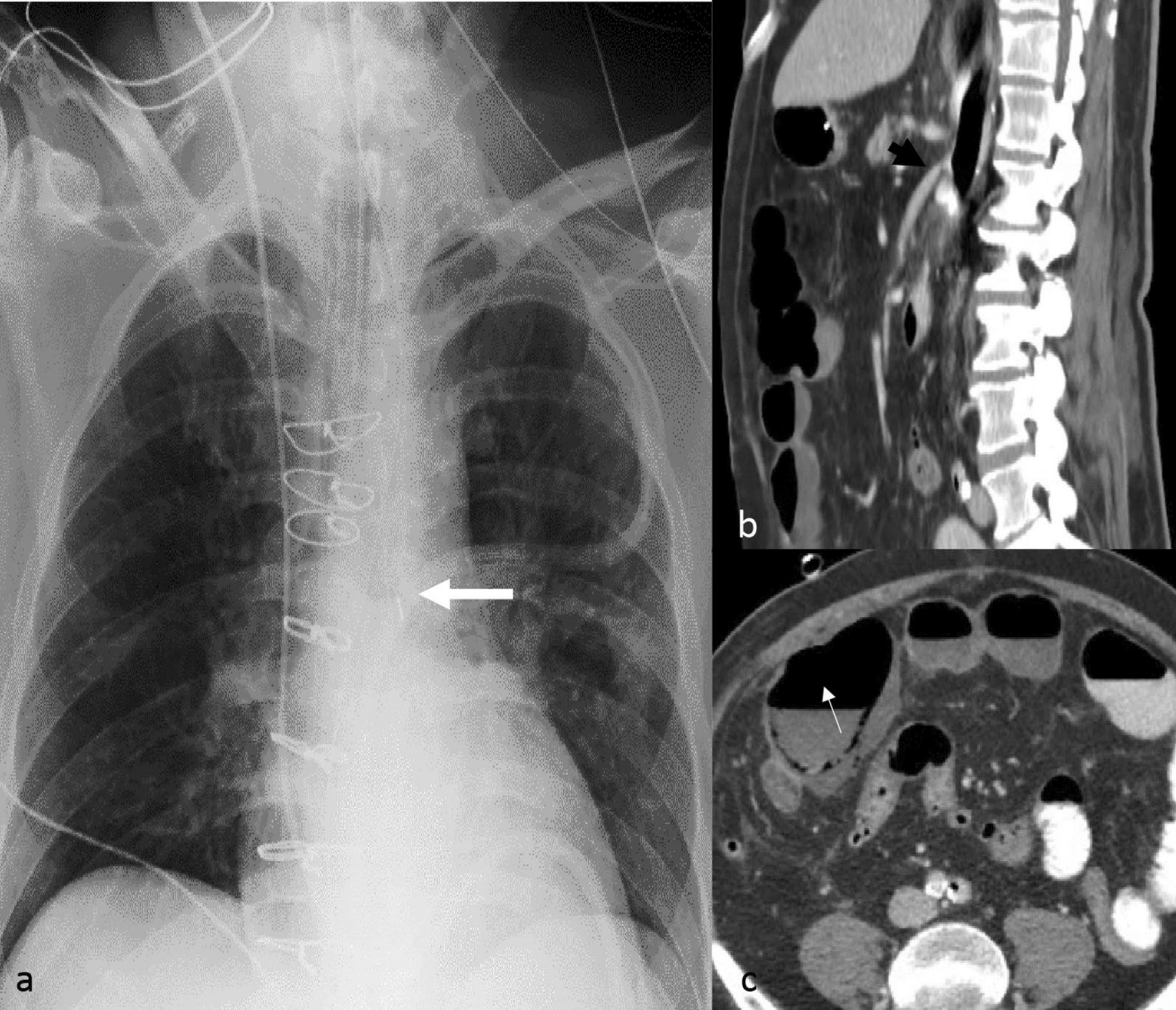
Complication from low IABP. AP chest radiograph (a) of a patient with an intra-aortic balloon pump. The upper radiopaque marker is below the carina (white arrow) which suggests a low position. Sagittal image (b) of a contrast enhanced chest and abdomen CT at the level of the celiac trunk and superior mesenteric artery (SMA) origin demonstrates high grade obstruction of the SMA and celiac trunk ostia by the distended IABP. Axial CT image (c) identifies complication from hypoperfusion: pneumatosis and contained perforation of the cecum (white arrow), due to the hypoperfusion from the high grade celiac trunk and SMA ostial occlusion. (IABP—Intra-aortic balloon pump)
